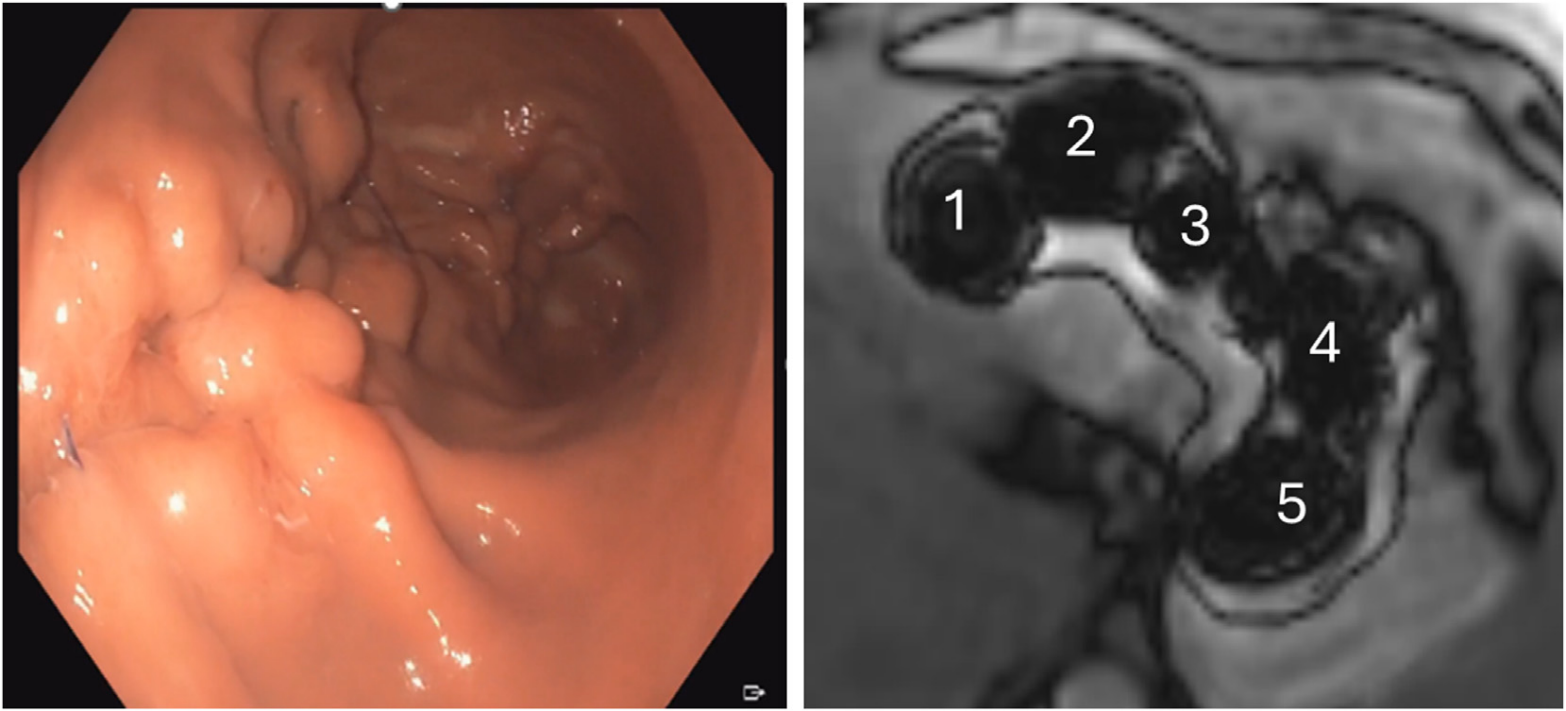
Endoscopic and magnetic resonance imaging evaluation of the iESG at 6 months demonstrating full retention of all sutures, robust full-thickness plications, and maintenance of the endoscopic sleeve effect. *iESG*, Interlocking endoscopic sleeve gastroplasty.
